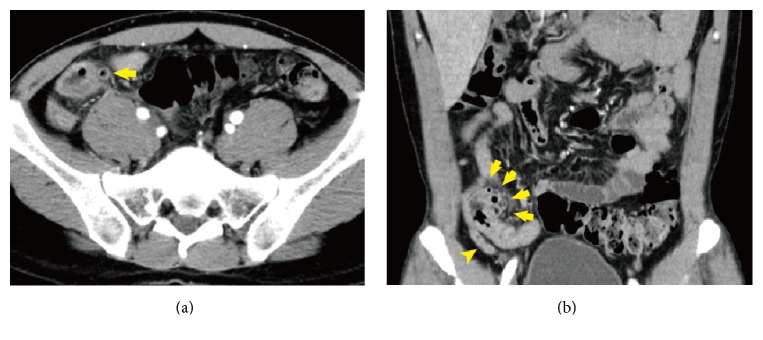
Computed tomography revealed diverticula sequentially located in the wall thickening terminal ileum (arrows); surrounding abdominal fat was developing high density suggesting inflammation. The appendix was not swollen (arrow head).
